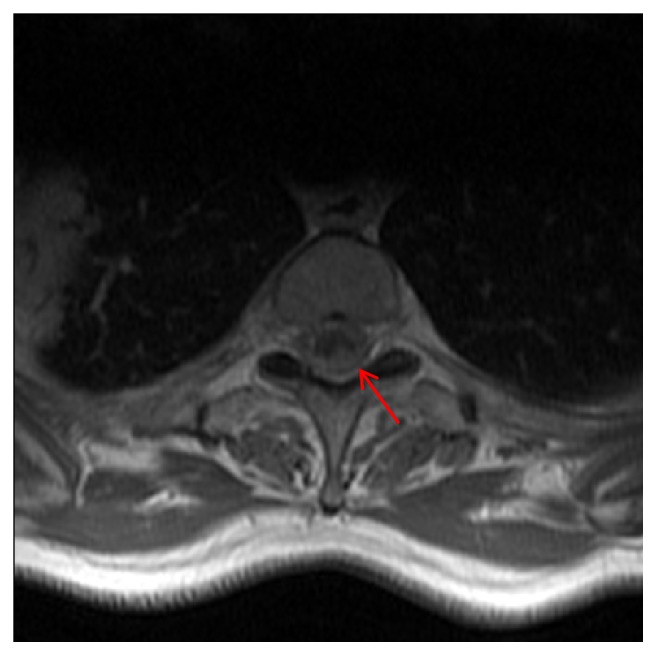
Axial gadolinium-enhanced T1-weighted Turbo Spin Echo MR image. An enhancing mass surrounds, deforms, and compresses the spinal cord all around its perimeter but most exuberantly in the left posterior side (red arrow).
